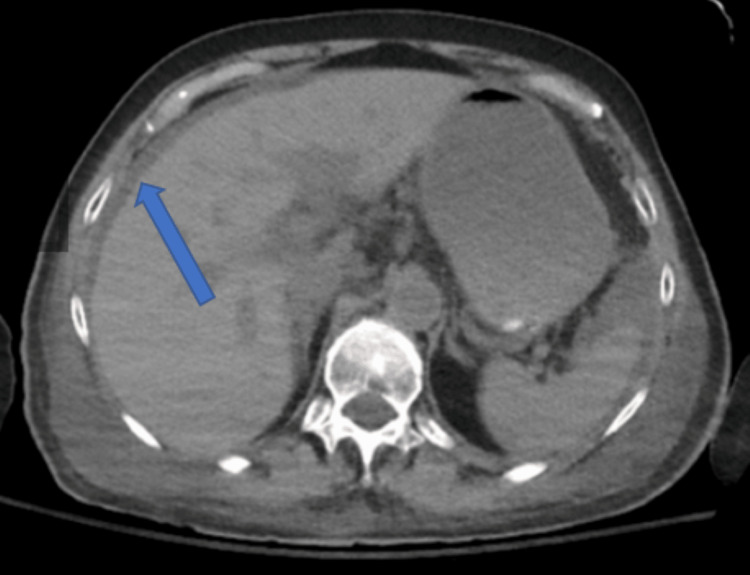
CT without contrast of the abdomen/pelvis (transverse view). Radiologic impression read as residual moderate intra-abdominal ascites.
